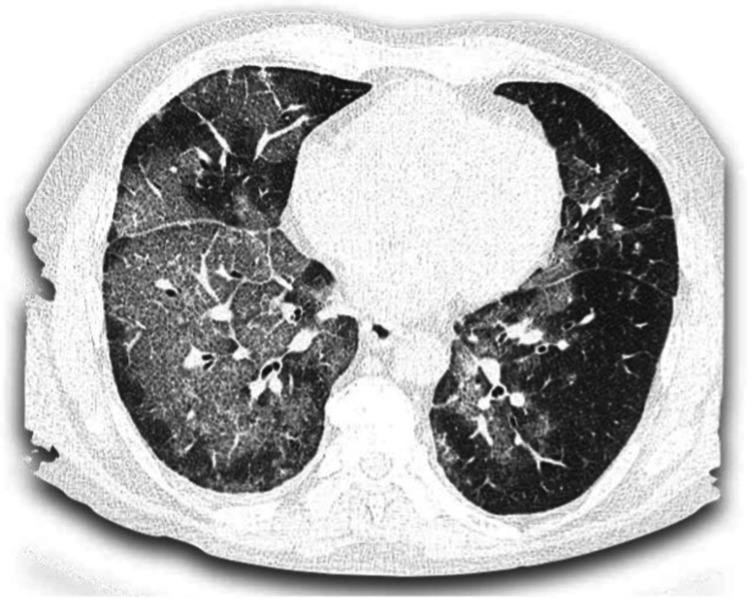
Axial nonenhanced chest CT images (lung window) show bilateral multiple ground-glass opacities
